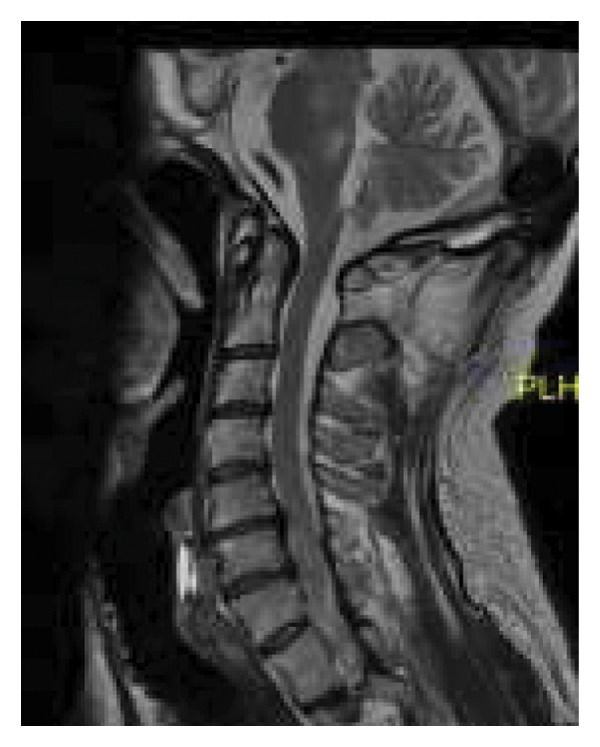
Cervical spinal MRI, T2 sagittal view. Hyperintense image at C5–C6.
